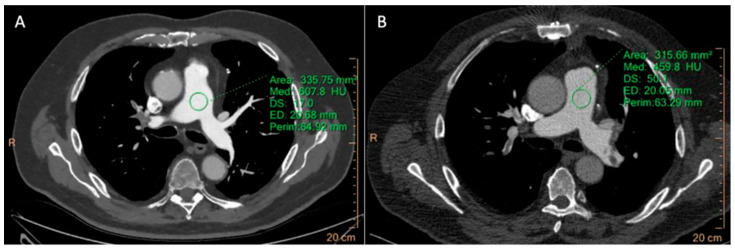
Two 65-year-old (A) and 71-year-old (B) men both underwent 256-row CT pulmonary angiography study, showing pulmonary embolism. The MBIR algorithm (A) shows a higher attenuation value of 608 HU with a lower noise value of 17 HU compared to HIR (B) reconstruction (460 ± 50 HU).
